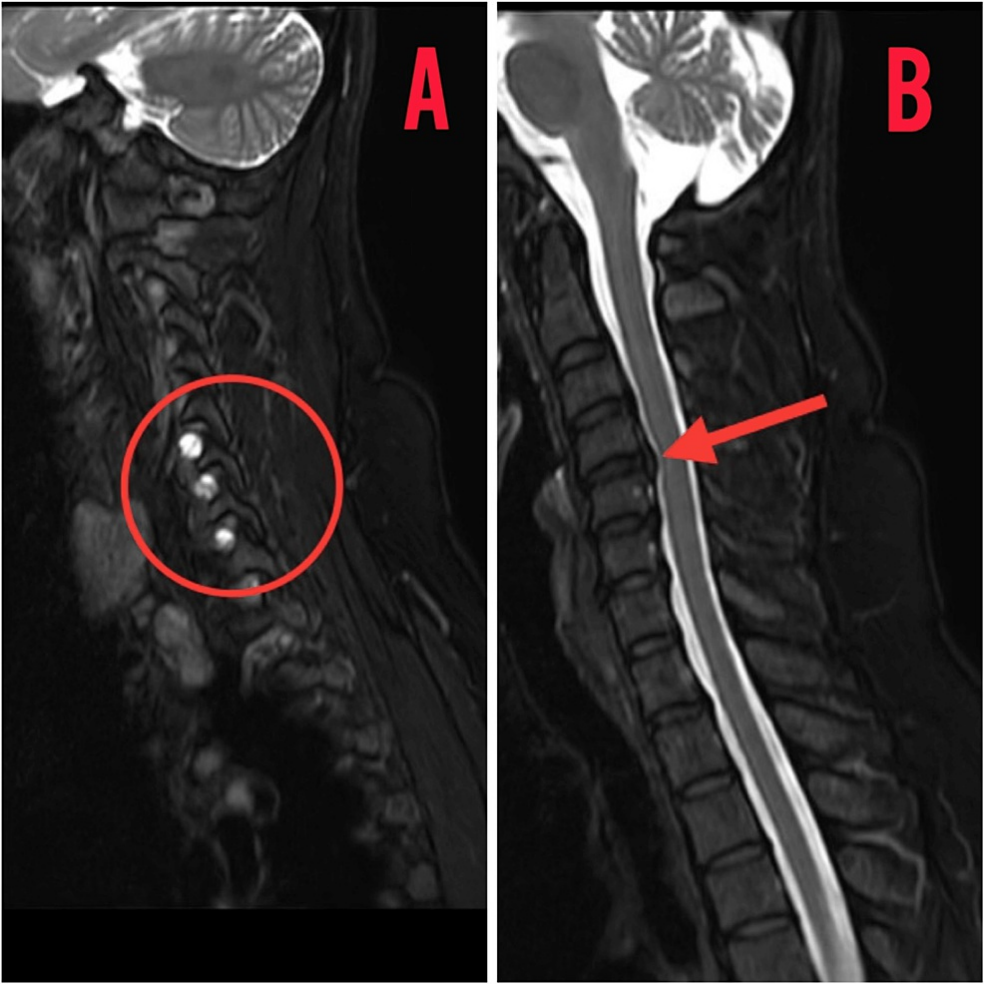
MRI of the cervical spine in the sagittal view showing hyperintense shadows of cyst and bulging of discs MRI: Magnetic resonance imaging (A) Hyperintense lesions over C4,C5,C6 vertebrae; (B) disc bulging over C3-C4, C4-C5, C5-C6, C6-C7 vertebrae levels
